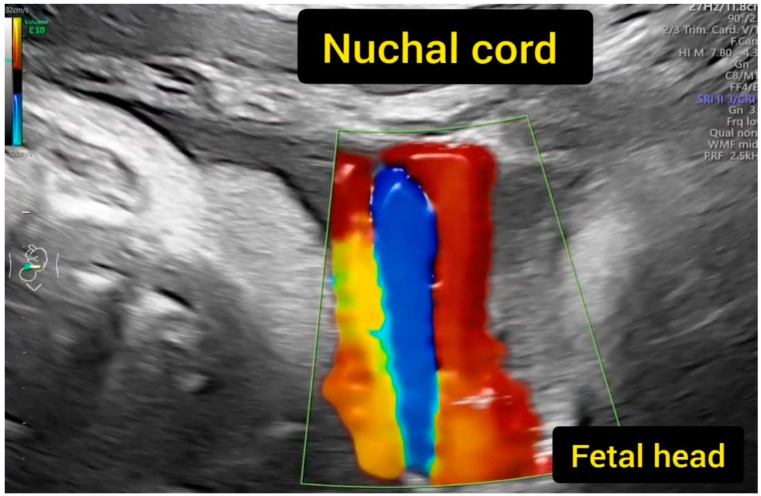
Status evaluation of the umbilical cord around the fetal neck with color Doppler.
